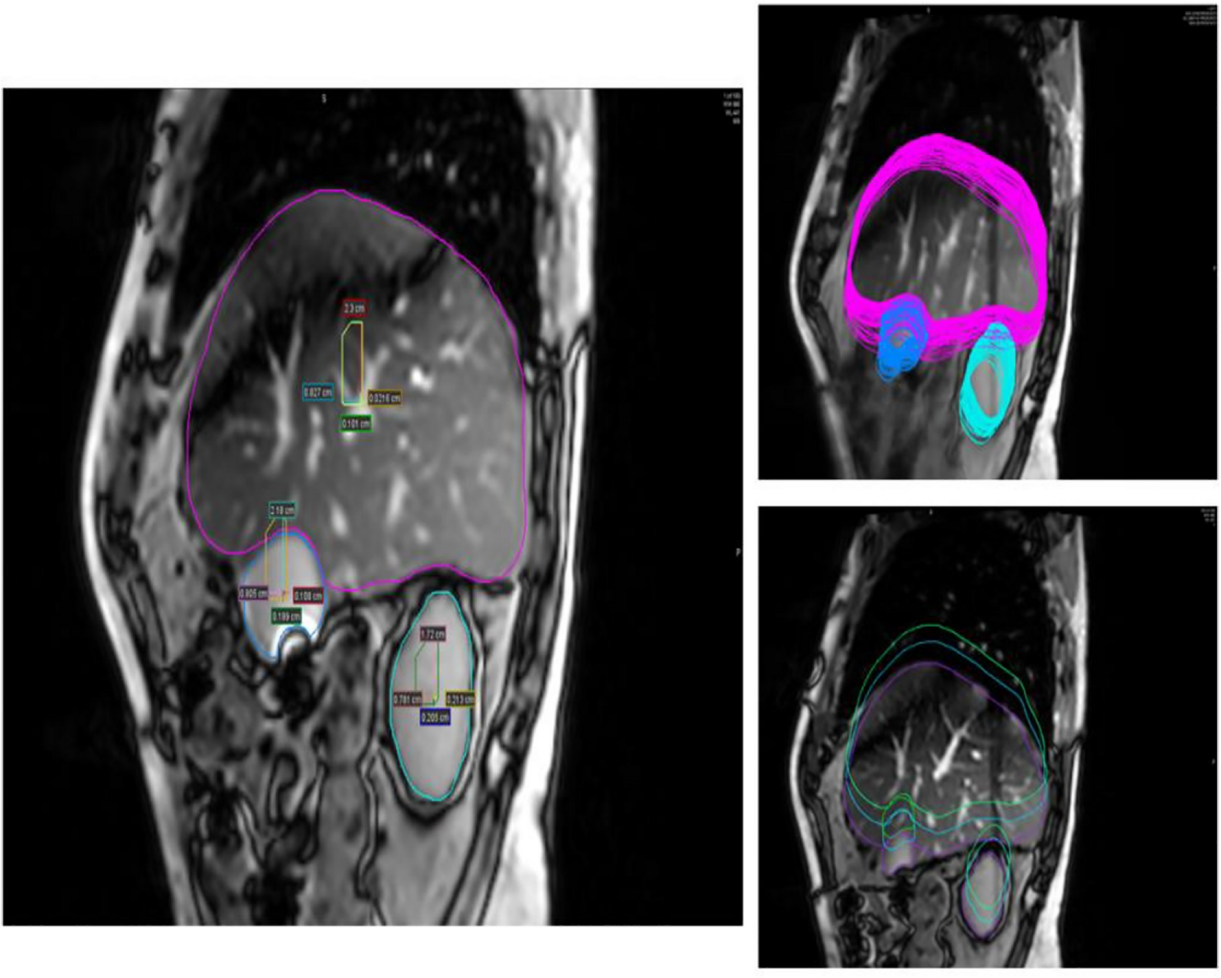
Liver, GB and kidney motions in 100 seconds using 2D cine-MRI in SI and AP directions. Center of mass motions of liver and GB in both directions (left). Maximum and minimum motion of liver and GB due to respiratory (right).
